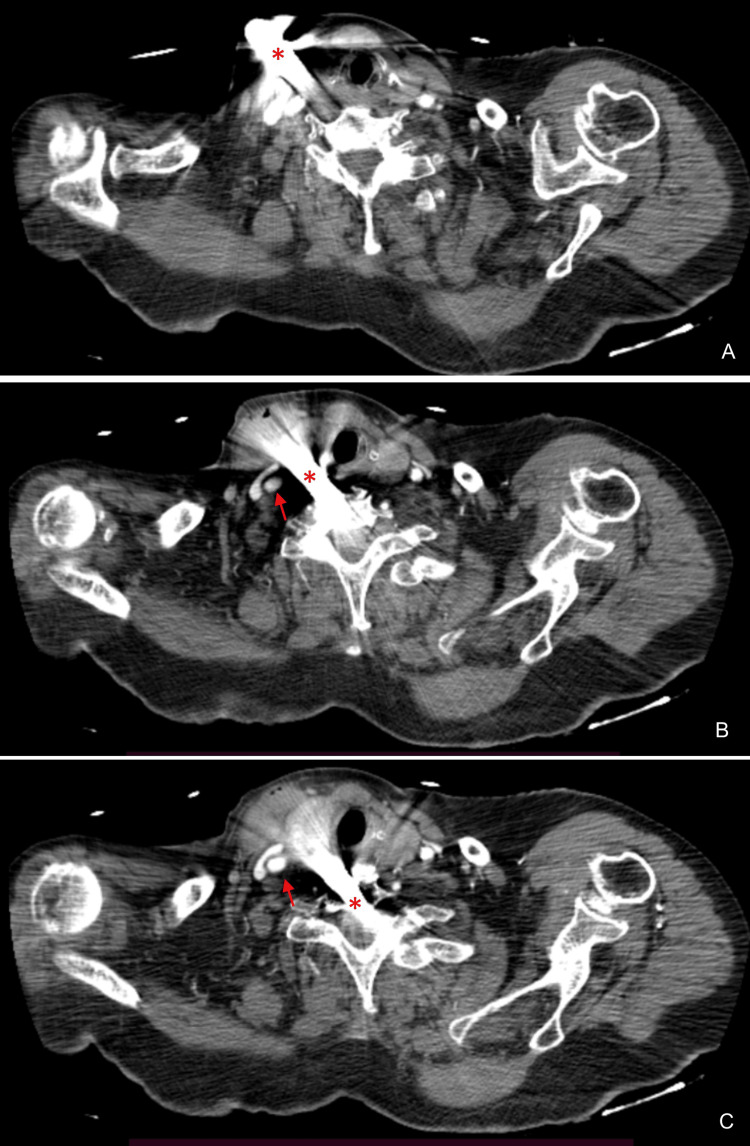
Axial enhanced computed tomography (CT) acquisitions showing a penetrating metallic object (red asterisk) at the level of the right laterocervical region The object had an inferomedial path, traveling immediately tangential to the anteromedial side of the right common carotid artery (red arrow), which remained patent and showed no signs of laceration. The metallic blade was also adjacent to the right lobe of the thyroid and penetrated through the C6-C7 intervertebral space, piercing the C7 vertebral body.
